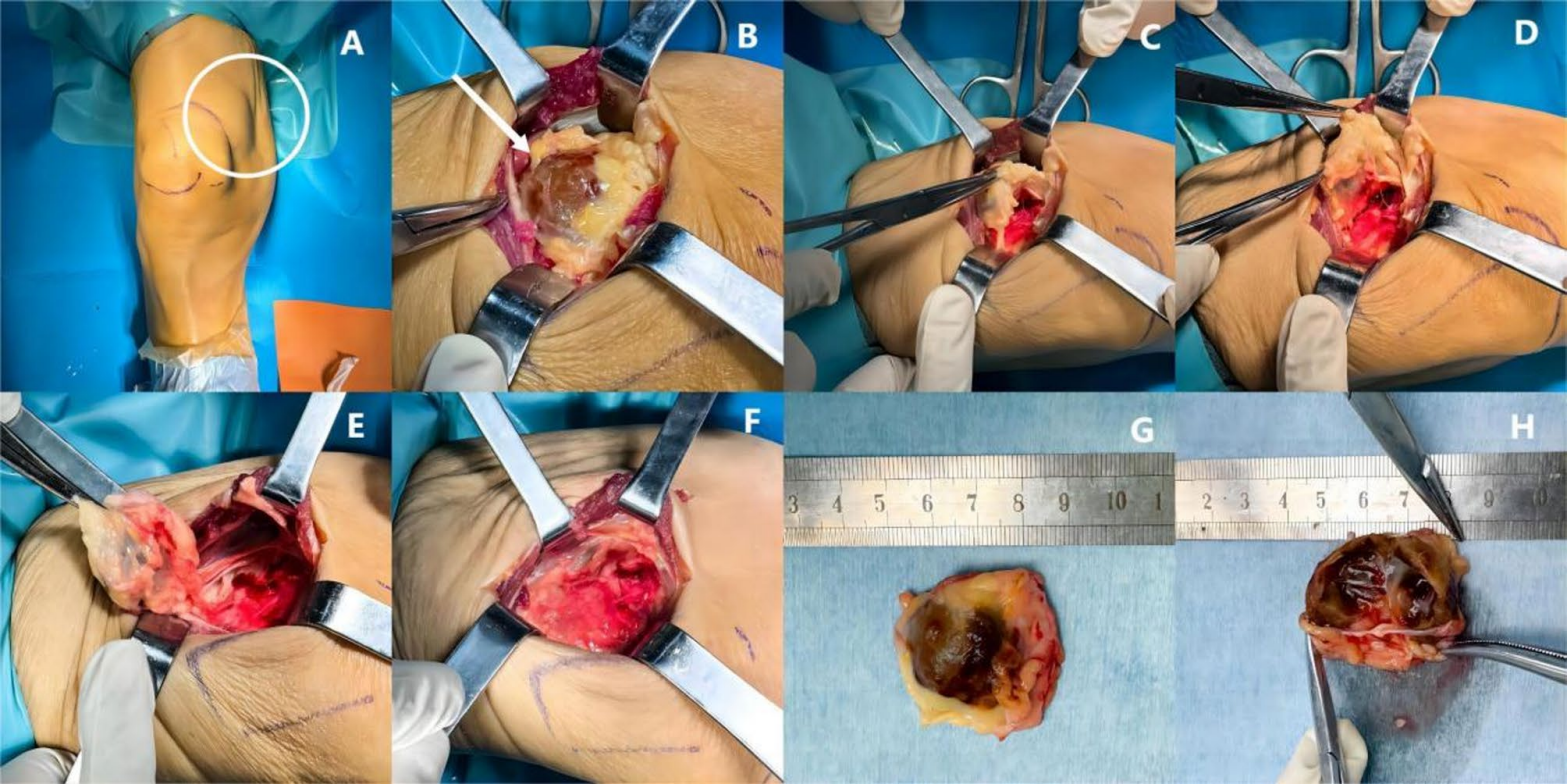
Intraoperative conditions. A The mass was palpated. The lesion was noted as being located at the right suprapatellar bursa based on the preoperative PET-CT results. B Cut layer by layer through the skin to reveal the medial femoral muscle and subcutaneous tissue, and then follow the muscle’s outline to the tumor. C, D, E Total dismantling of the mass and a rough division of the mass along the edges. E The mass was entirely removed. The wound was irrigated. G, H After the mass was entirely separated, a brownish-brown fluid spilled out of it. The mass measured approximately 2.7 * 2.5 * 1.4 cm and had a smooth surface, a dark red color, and a firm texture
